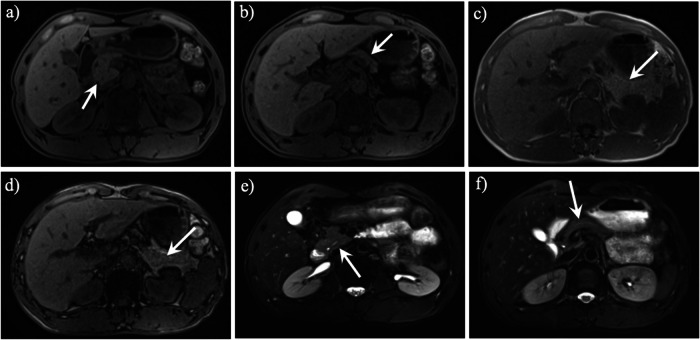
A 48-year-old man with partial dorsal pancreatic agenesis. Axial fat-suppressed T1-weighted MR images (a, b) revealed a normal-appearing pancreatic uncinate process, head (a, arrow), neck (b, arrow), with the body-tail portion partially absent. The in-phase (c) and out-of-phase (d) T1-weighted MR images revealed that the partial absence of the pancreatic body-tail portion was filled by the adjacent retroperitoneal fat (arrow). Axial T2-weighted MR images (e, f) showed only the pancreatic duct in the head, neck and partial body of the pancreas with linear high signal (arrow), but the pancreatic duct of the tail was invisible
